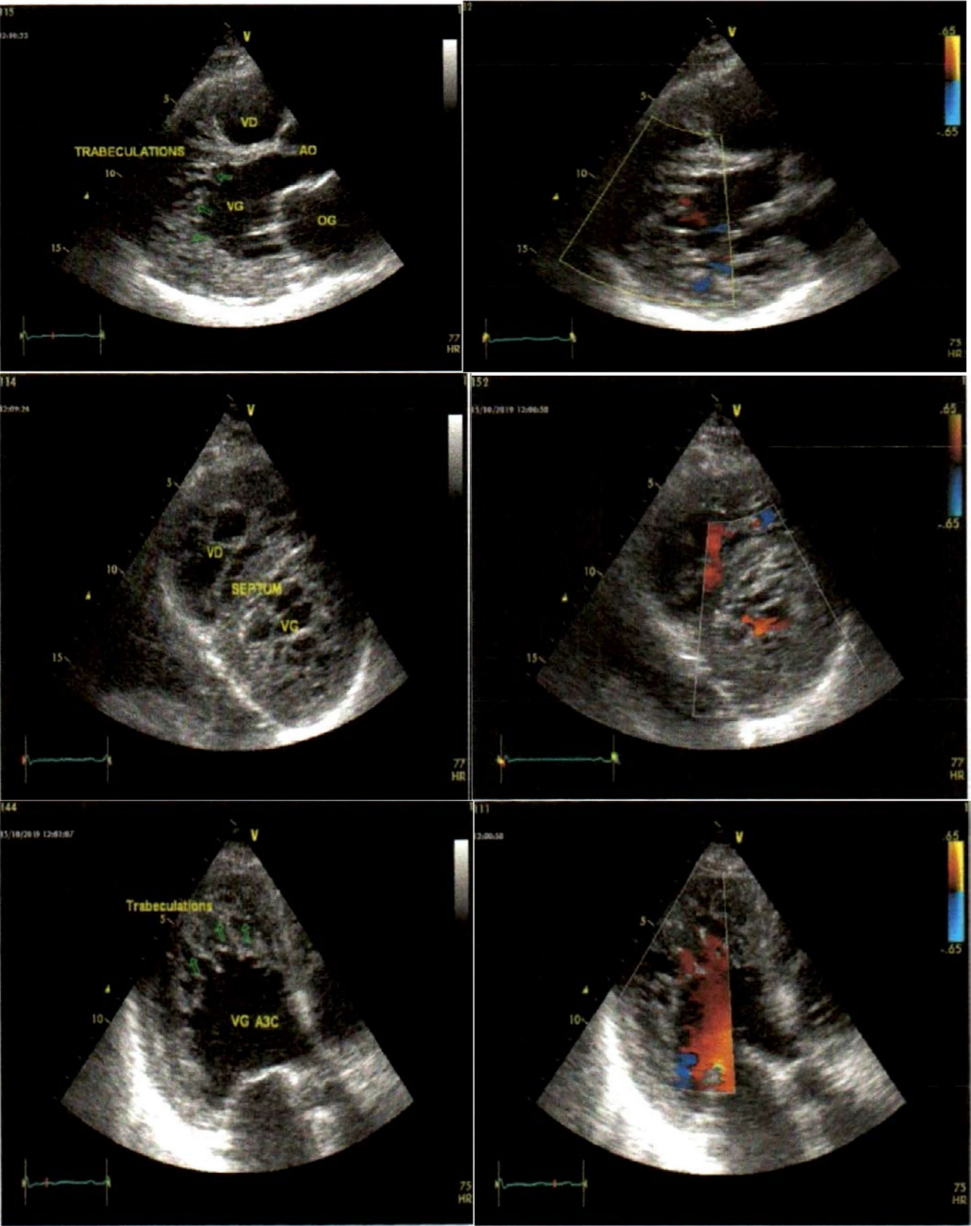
2D echocardiogram of the left ventricle. Upper panel shows the parasternal long axis with prominent trabeculations with Doppler within the recesses. The middle panel shows the parasternal short‐axis view of both ventricles with prominent trabeculations and intrarecess Doppler. The lower panel shows the apical 3‐chamber view of the left ventricle with prominent trabeculations and intratrabecular blood flow
